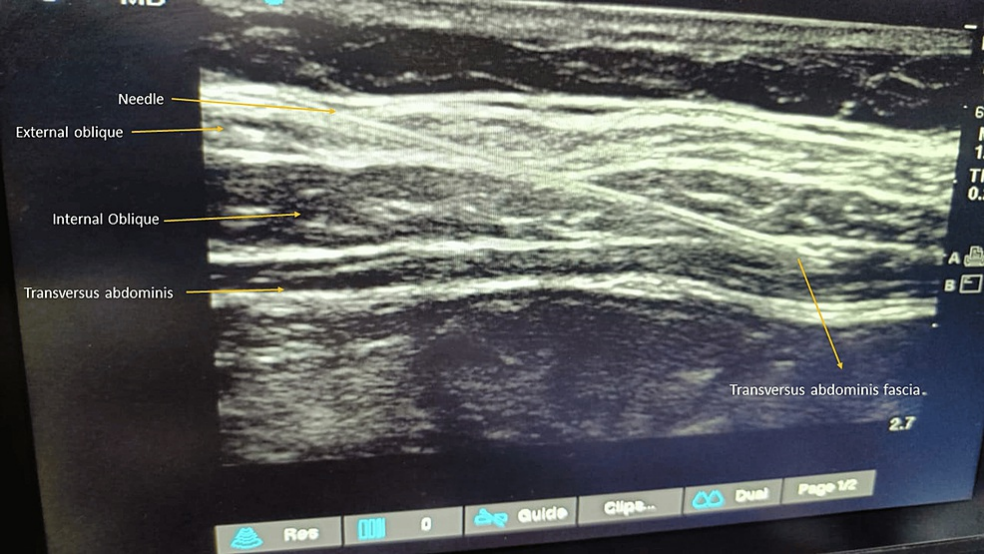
Ultrasound image of the TAP block TAP: transversus abdominis plane
